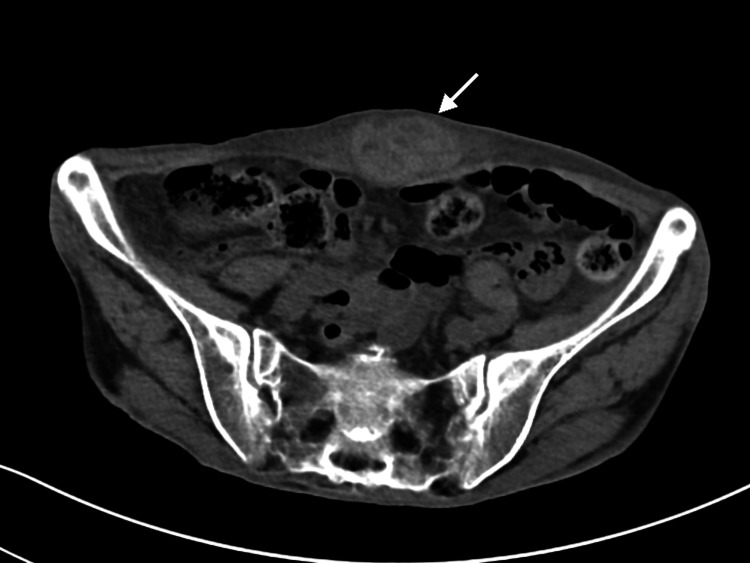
Computed tomography image Abdominal and pelvic computed tomography image shows an 8 cm × 5 cm × 3.5 cm heterogeneous area with relatively high attenuation within the rectus sheath musculature (arrow) consistent with a rectus sheath hematoma. The normal rectus muscle appears uniform and does not have such collections.
